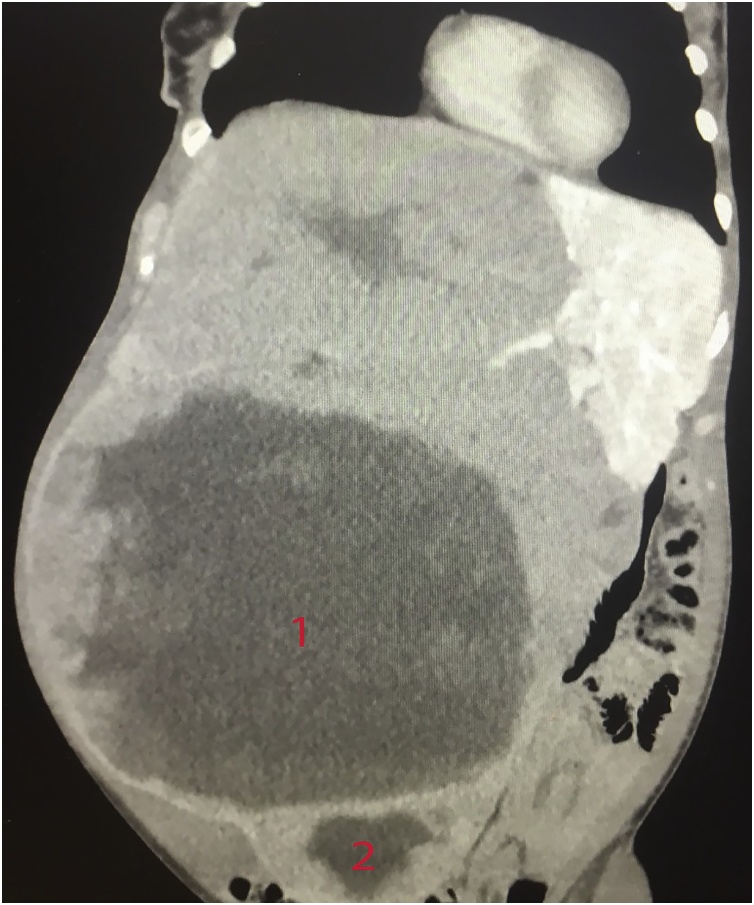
Pre-operative computed tomography scan of liver showing two huge hypodense masses as labeled 1 and 2.
